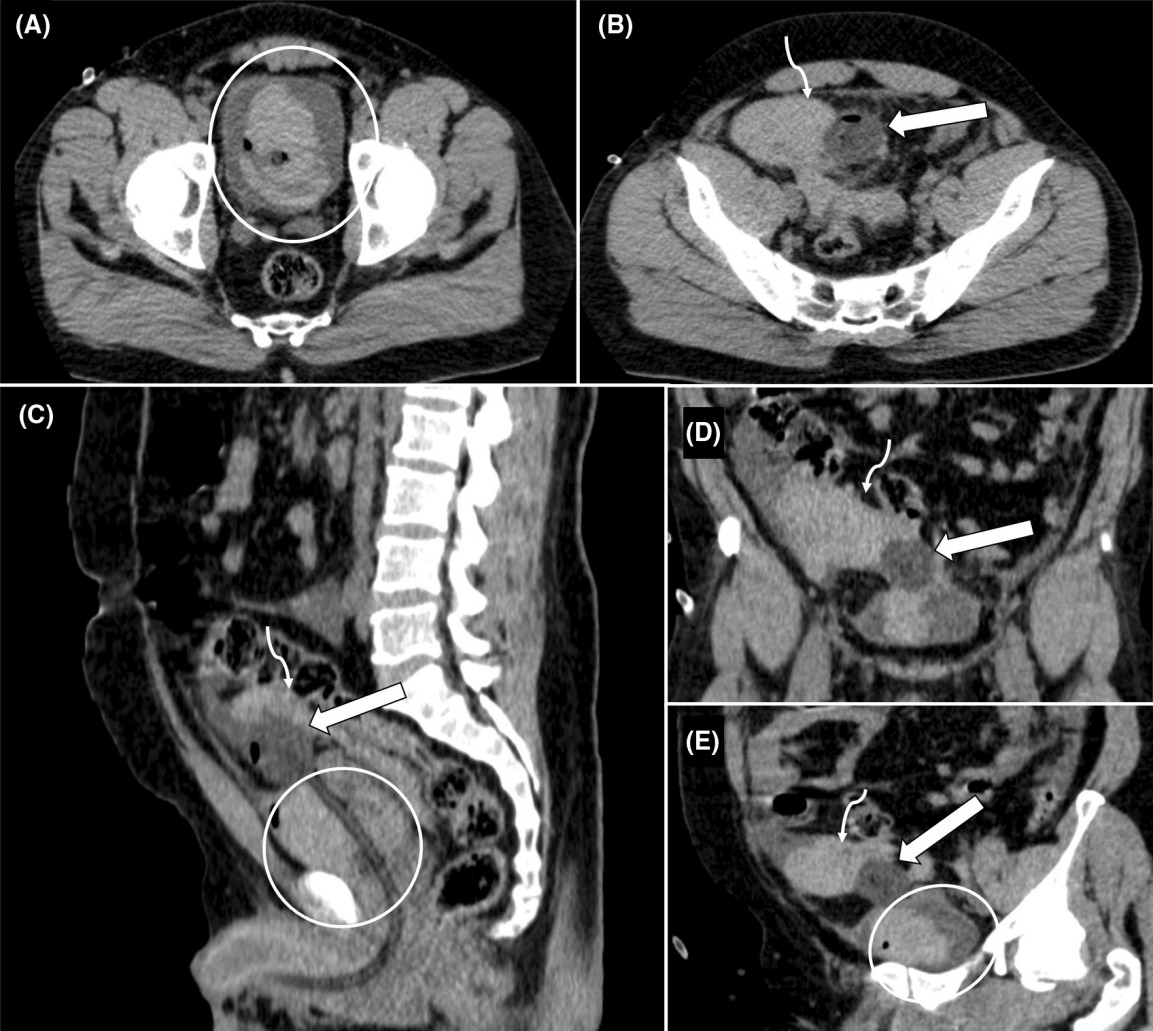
Axial (A, B), sagittal reconstructed (C), coronal reconstructed (D), and coronal oblique reconstructed (E) images of non‐contrast CT showing urinary bladder hematoma (white circles) with foley's bulb (white arrows) lying outside urinary bladder traversing through the dome and surrounding intraperitoneal hematoma (curved arrows).
